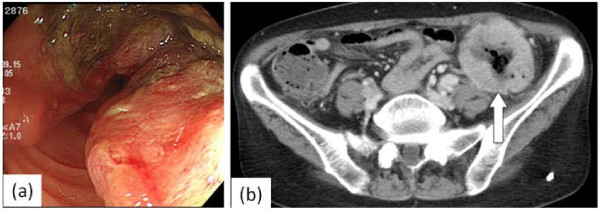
Colonoscopy discloses bulky disease with stricture in the sigmoid colon. Abdominal computed tomography scan reveals sigmoid colon tumor invading the abdominal wall unaccompanied by swelling of colonic lymph nodes and distant metastasis.
